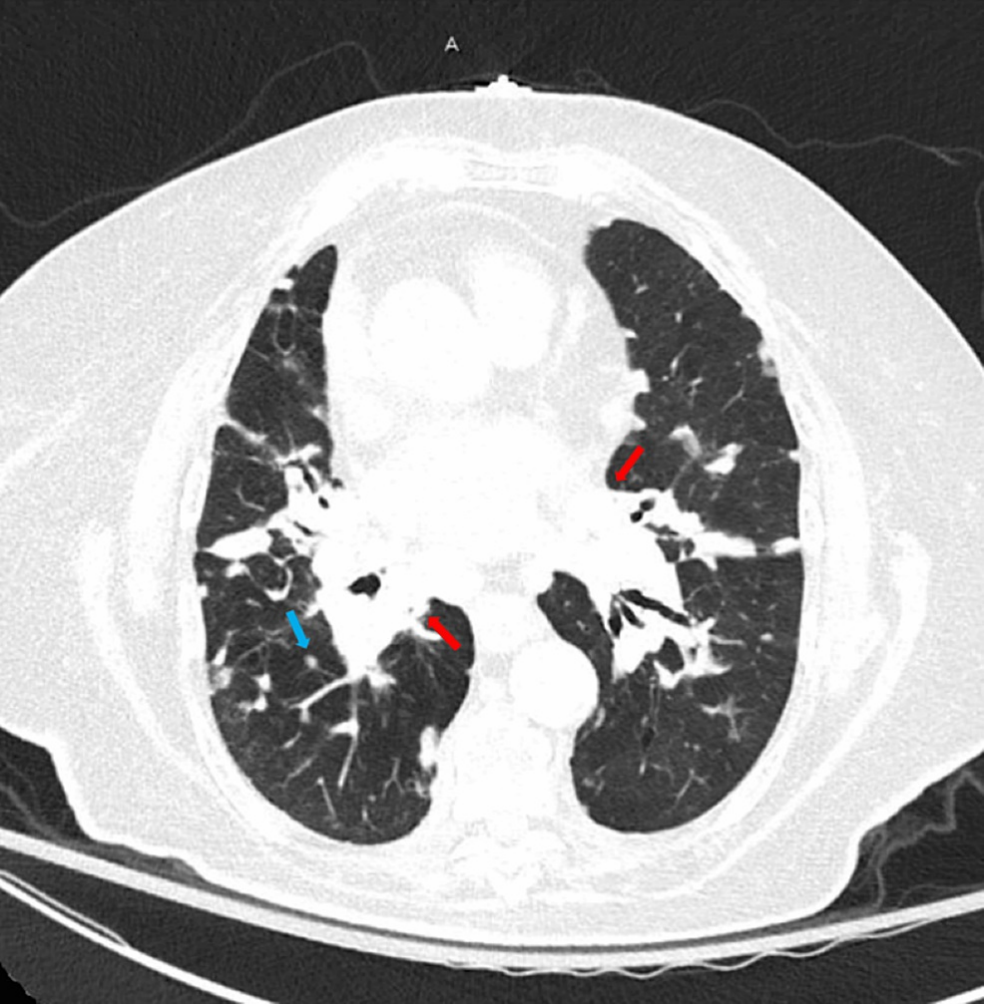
Chest computed tomography without contrast showing moderate peribranchial vascular nodularity (blue arrow) and extensive calcified mediastinal and bilateral hilar adenopathy (red arrows) unchanged from previous imaging and consistent with stable sarcoidosis
